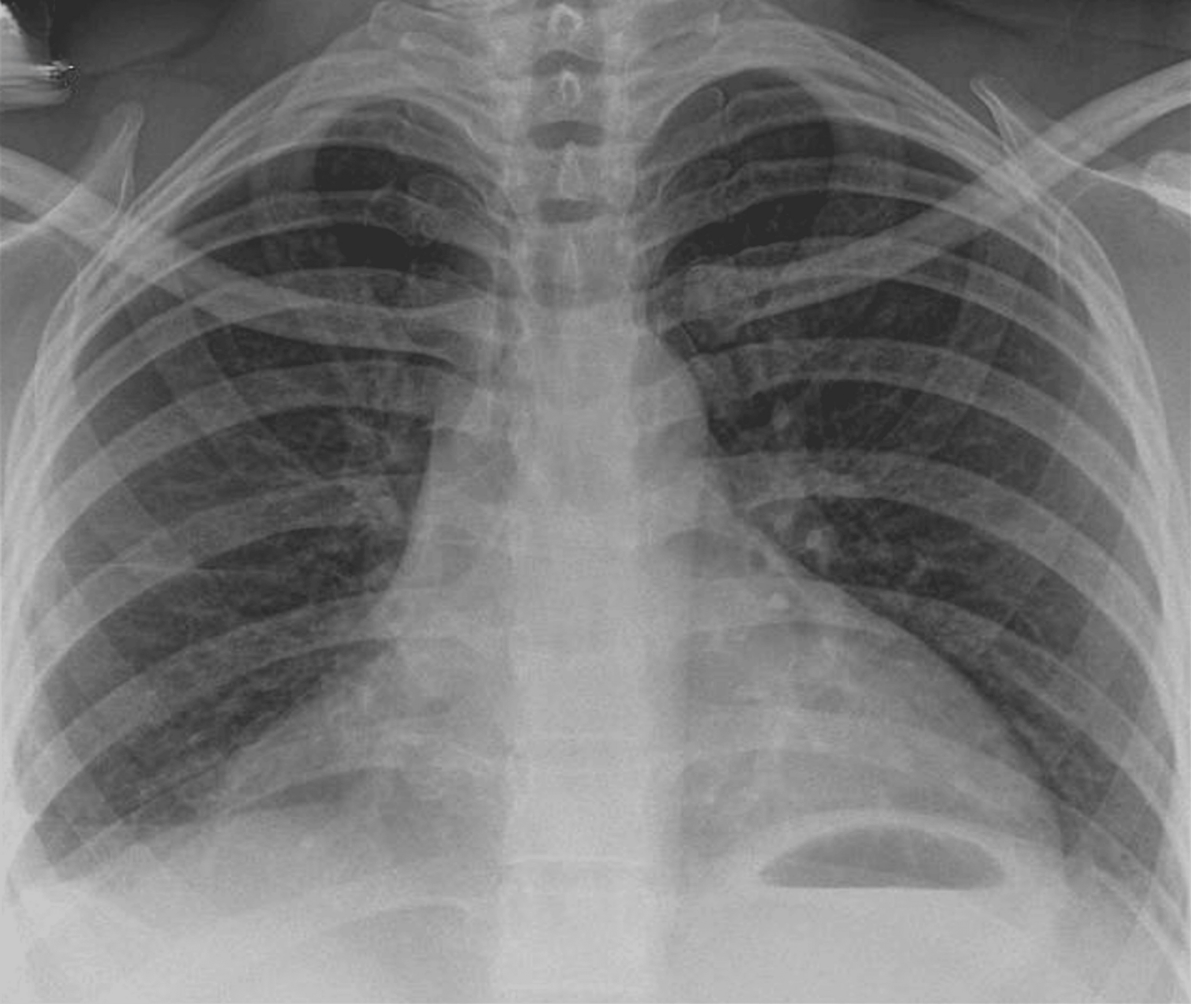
Chest X-ray showing resolution of pleural effusion following colchicine therapy
